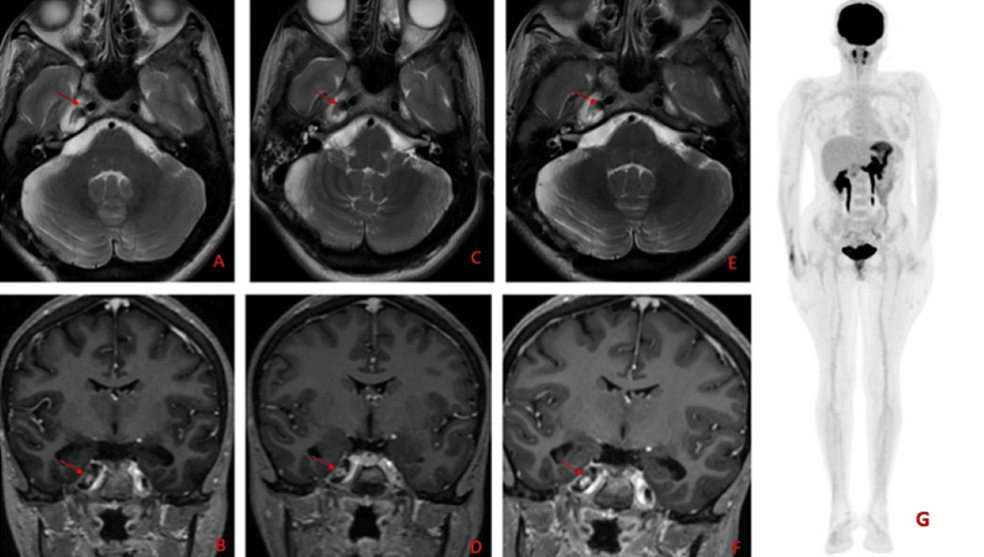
Serial MRI brain done on follow-ups at three months (images A and B) six months (images C and D) nine months (images E and F) showing stable disease marked by red arrows and PET scan at 12 months showing no evidence of distant metastasis (image G)
